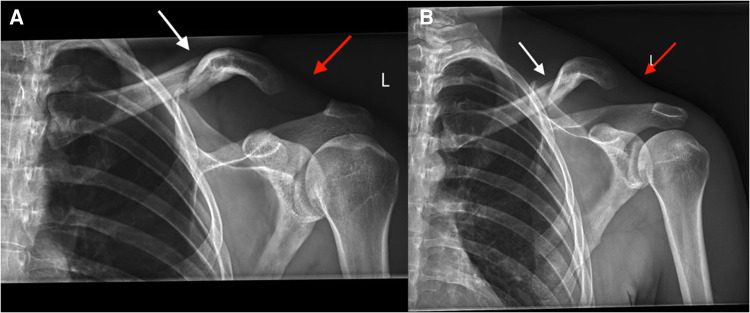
Radiographs demonstrating midshaft clavicle fracture with associated acromioclavicular joint dislocation (white arrow demonstrating the fracture site, red arrow demonstrating the dislocation site).
